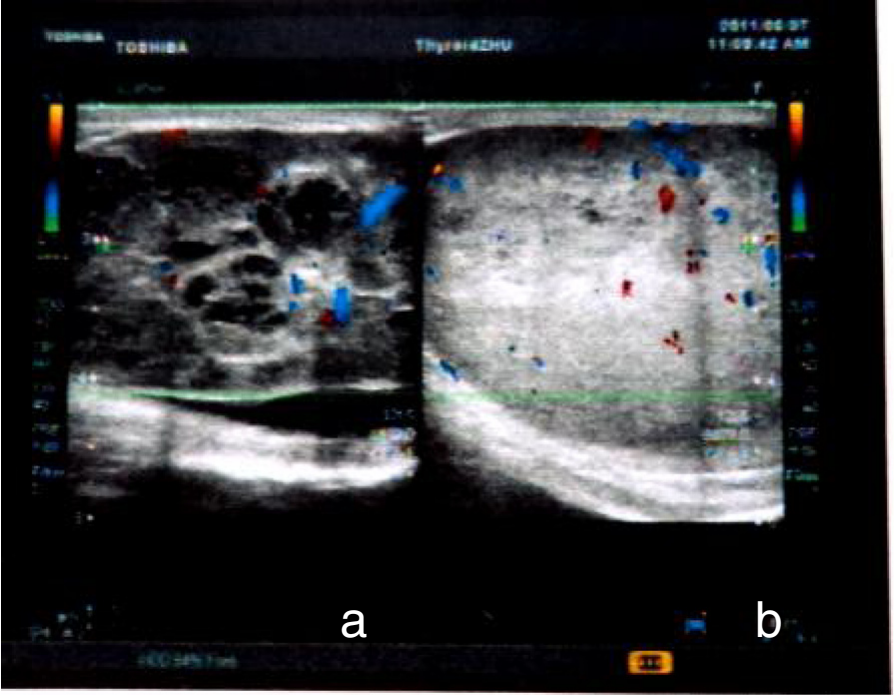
Scrotal ultrasonography of bilateral SS. (a) A left testicular multilocular tumor, (b) a right testicular solid tumor.
